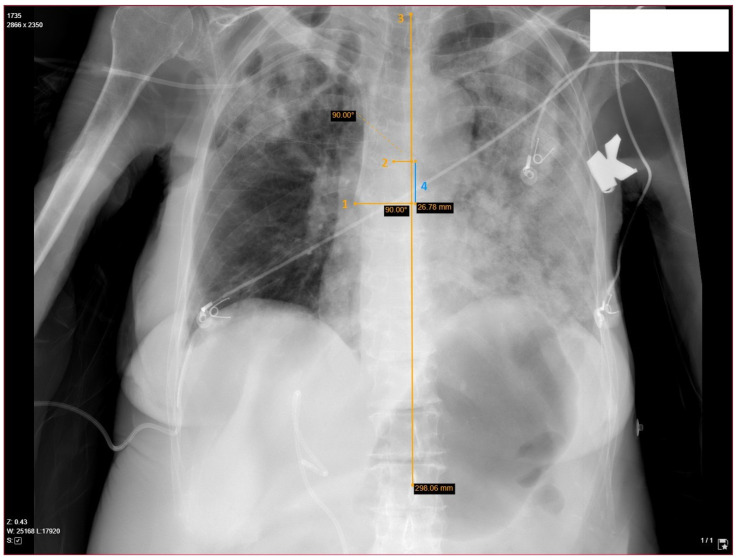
Catheter’s tip confirmation and optimal insertion length measurement demonstrated with yellow markings. Chest X-ray in the supine position obtained after surgery: after determining the patient’s axis using 1st and 12th thoracic vertebrae as landmarks (3), the distance between the carina and catheter’s tip are measured on the axis (4), which is subtracted or added to 20 cm (default insertion length in this study). 1—catheter’s tip, 2—carina, 3—patient’s axial axis, 4—catheter’s tip shift from the ideal depth.
